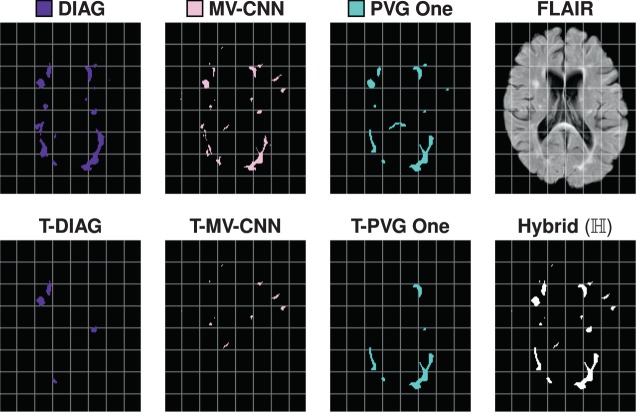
For a test data set, in the top row, we show the axial slices of the DIAG, MV-CNN, and PVG One segmentations, and the corresponding FLAIR image. In the second row, we show the volume thresholded version of DIAG (T-DIAG), MV-CNN (T-MV-CNN), and PVG One (T-PVG One), after the corresponding thresholds have been applied from the 2-Fold variety of our hybrid algorithm. The final image in the second row is the segmentation generated from the union of these results and is denoted Hybrid ($${\mathbb{H}}$$). For this subject, the IMI algorithm did not contribute any lesions and the corresponding images are not displayed.
